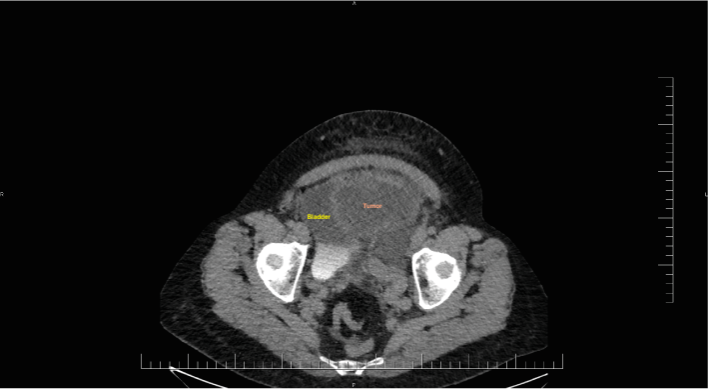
Axial CT image showing the close anatomical relationship between the urinary bladder and the tumor.
